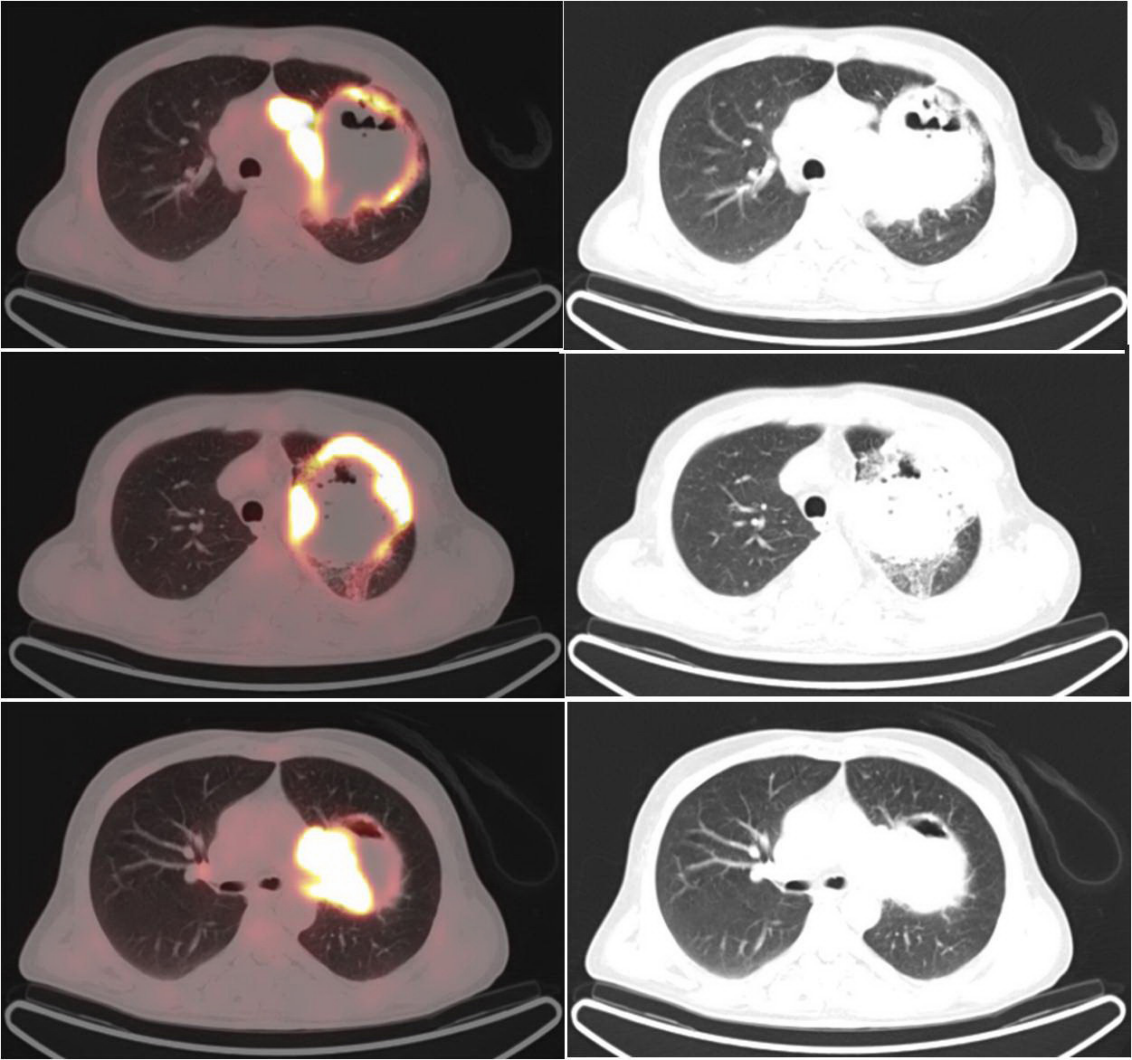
^18^FDG-PET/CT showing a heterogeneous mass on the left upper lobe surrounded with a rim of intense FDG uptake (SUV_max_=10.8) (There was no FDG uptake in the center of the mass. There were multiple mediastinal lymph nodes with a high FDG uptake in the aortic region with a SUV_max_ of 15.3.)
